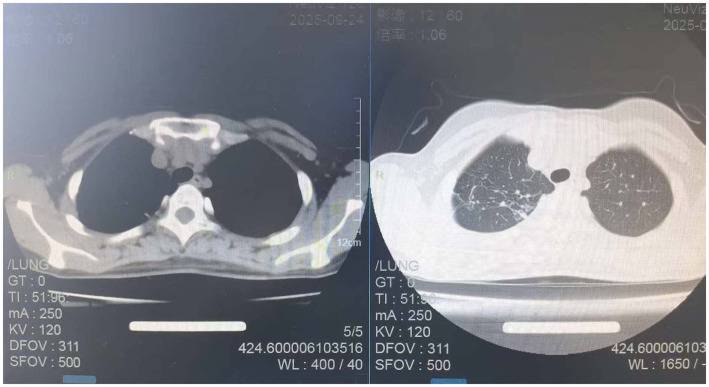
Follow-up chest CT demonstrating reduction in cavity size after adjusted anti-tuberculosis therapy.
